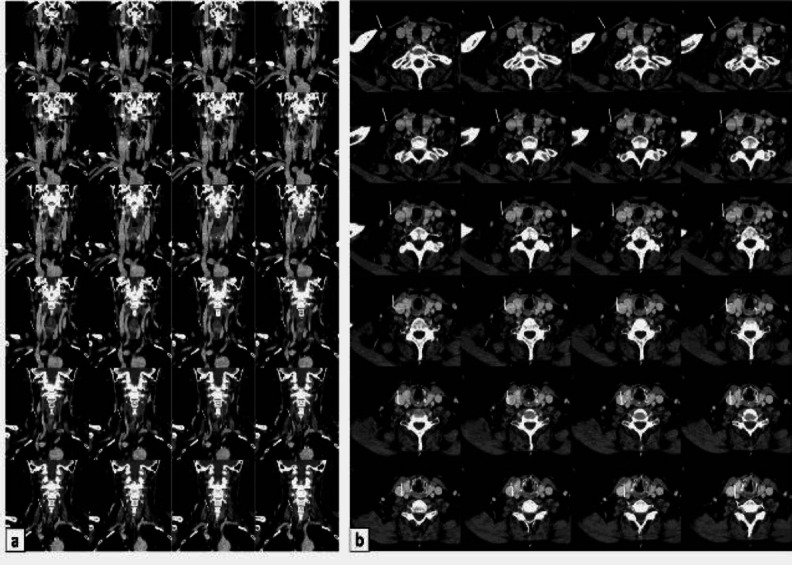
Panel showing the muscle on the contrasted enhanced CT scan. Coronal view (a) and axial view (b). White arrow indicates the cleido-vertebral muscle; asterisk the sixth cervical vertebrae’s transverse process
